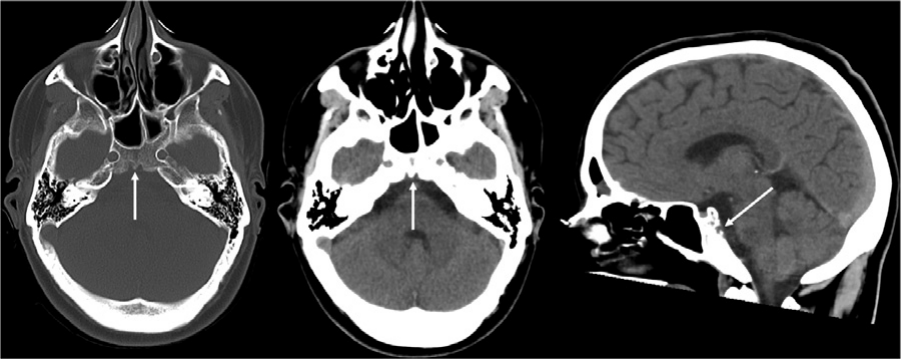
CT Brain without contrast showed an indeterminate lytic defect in the bony clivus with a well demarcated smoothly corticated margin (arrow). This was reported as concerning for malignancy, with recommendation for further evaluation with MRI brain and referral to our tertiary care center for further workup.
